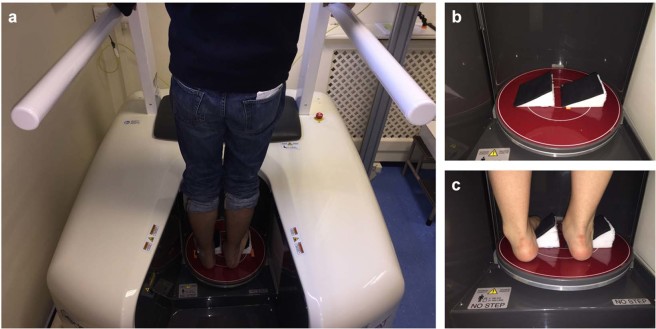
Bilateral weight-bearing PedCAT-CT. An X-ray source and a flat-panel detector on opposite sides rotates horizontally around the foot. (a) Subject positioned in bipedal standing position in pedCAT during scan. (b) Wedges placed in pedCAT platform to allow for eversion and inversion position of the feet. (c) Subject standing on the wedges with right foot in eversion and left foot in inversion configuration.
